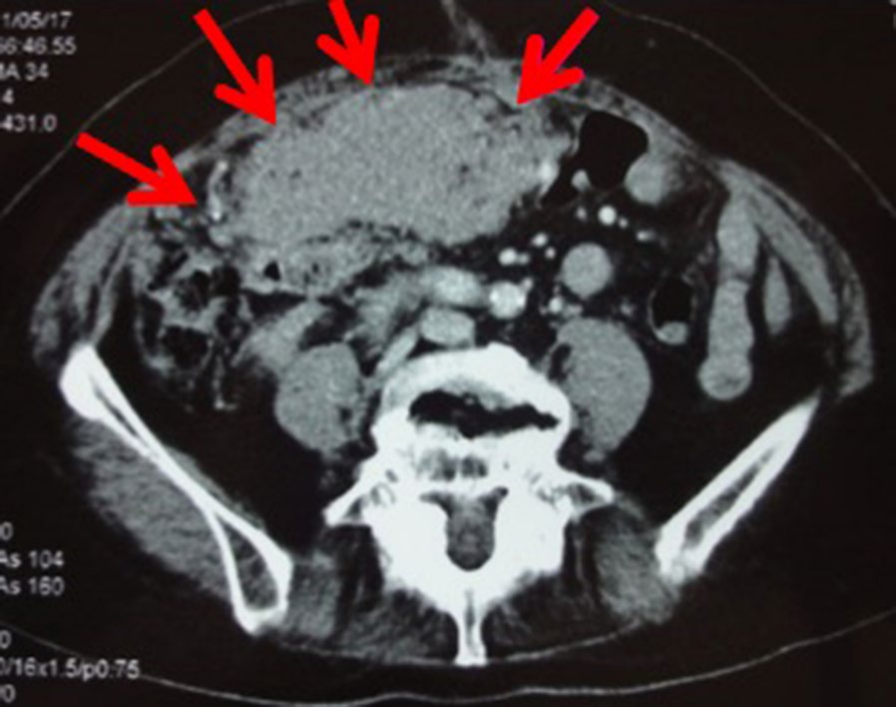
Preoperative findings by abdominal CT scan. A tumor about the size of one's palm was observed in the duodenum and the head of pancreas. The tumor was compressing the duodenum, causing loss of appetite and detention. Arrows (→) show the tumor’s margin occupying the abdominal cavity
